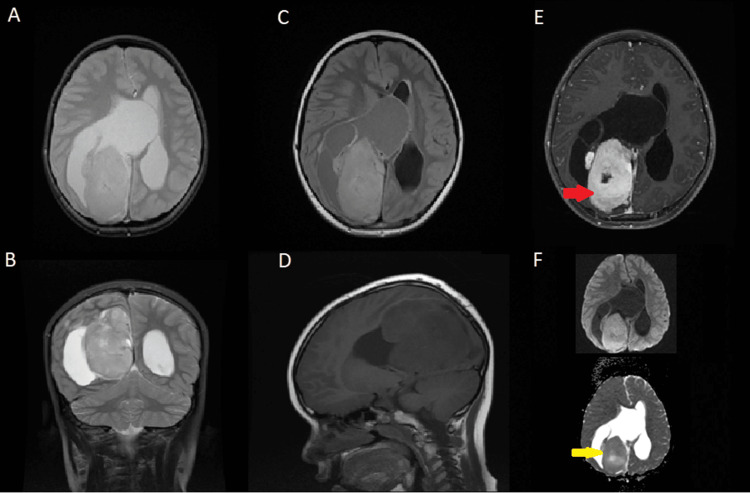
Axial (A) and coronal (B) T2-weighted images showing the solid-cystic component of the lesion with hyperintensity, centered in the parietal lobe, causing compression on the right lateral ventricle and midline deviation. Axial T2/FLAIR (C) reveals a decrease in signal intensity of the cystic component, which appears hyperintense compared to cerebrospinal fluid. Coronal T1 (D) demonstrates hypointense signal intensity on T1-weighted imaging. Axial post-contrast T1 (E) shows heterogeneous enhancement of the solid component (red arrow). DWI/ADC sequences (F) demonstrate iso-intensity of the ADC within the solid component, compared to the normal brain parenchyma (yellow arrow). FLAIR: fluid-attenuated inversion recovery; DWI: diffusion-weighted imaging; ADC: apparent diffusion coefficient
